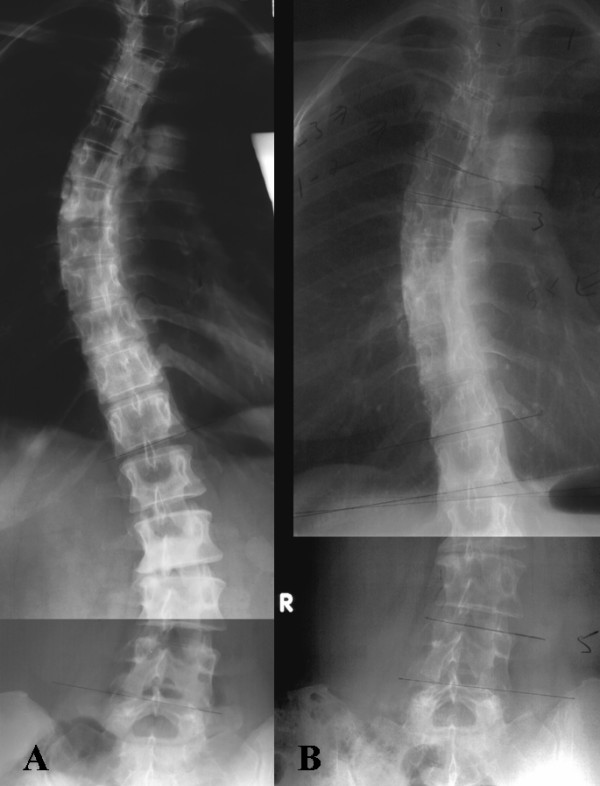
AP standing radiographs in (A) 1990 and (B) 2005.
